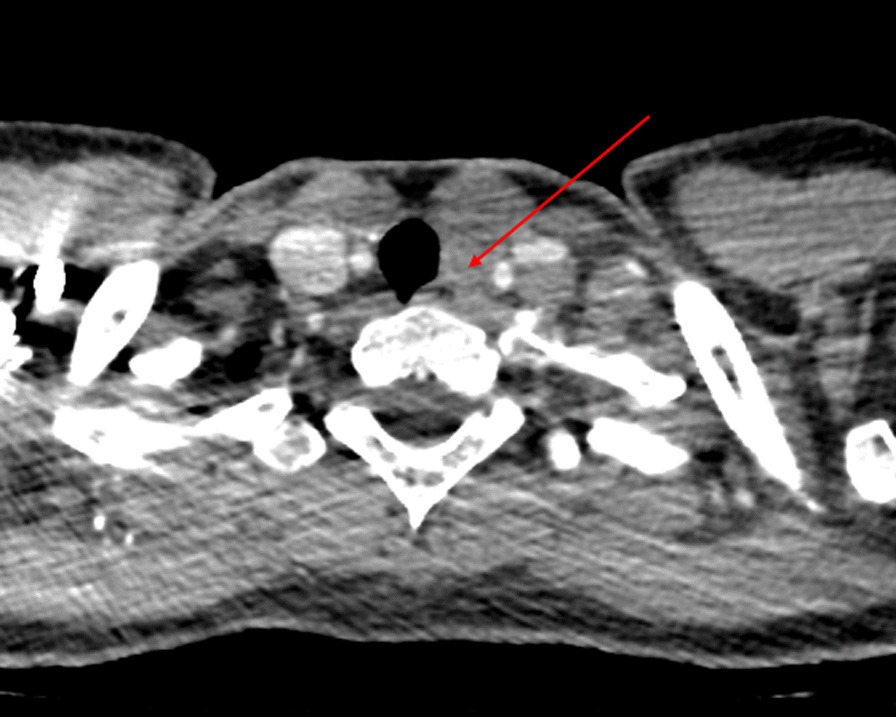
Neck CT scan showing a laterotracheal node of 4 cm proximate to the esophagus
